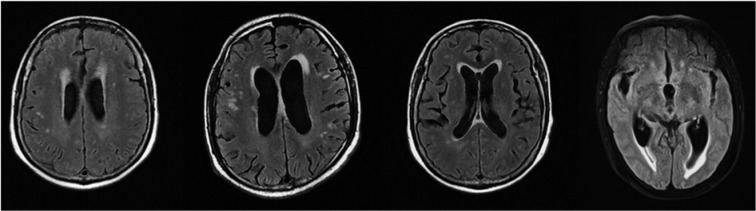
Representative MRI image of patients with ischemic foci and lacunar infarction in the brain after EGFR-TKI treatment
